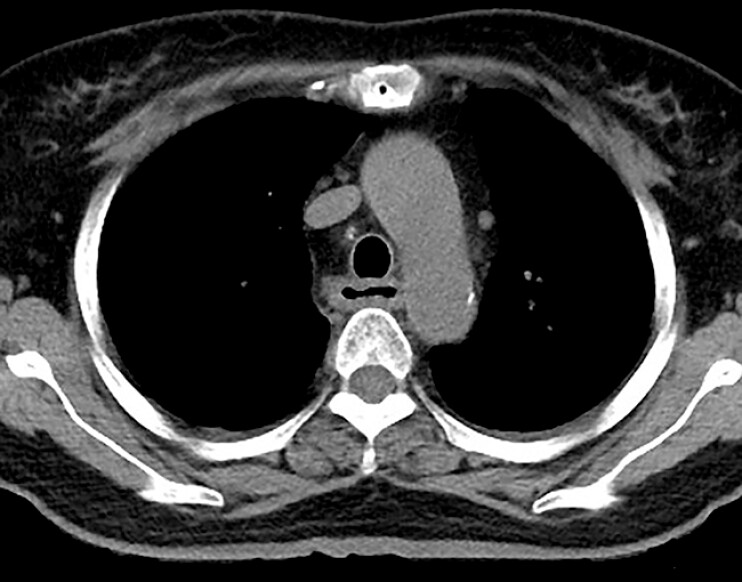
On day 55, computed tomography of the chest showed a significant reduction of esophageal wall thickening.
